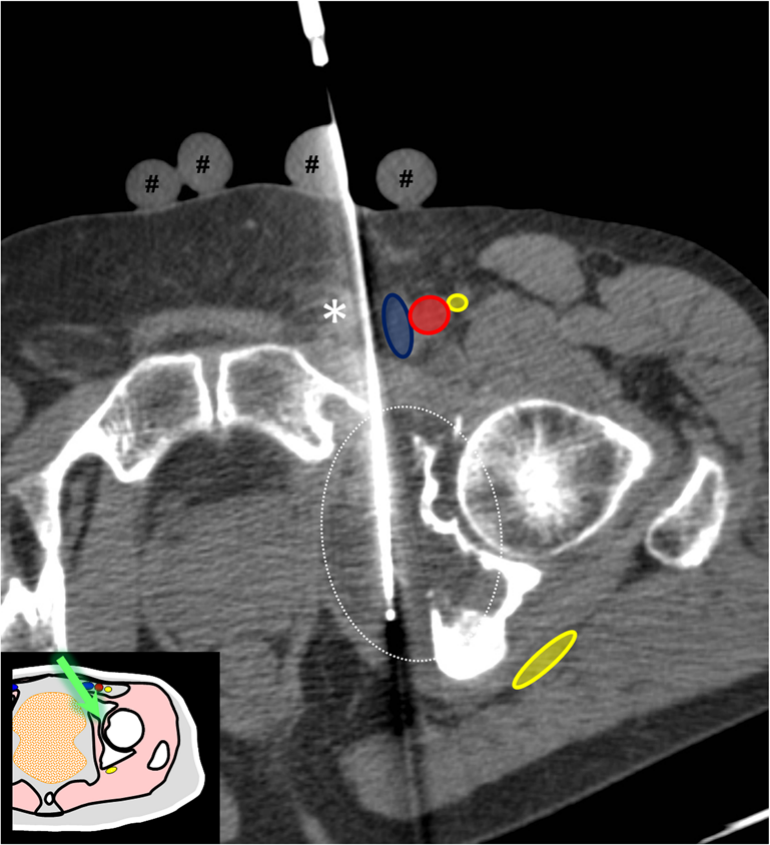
A 68-year-old man with bladder cancer presenting with painful acetabular metastasis. Axial CT shows one of the cryoablation probes inserted in the lesion with an approach between the spermatic cord (*asterisk*) and the femoral vein. The hypodense ice ball (*dotted line*) is seen. Note the glove fingers filled with warm saline over the skin to prevent thermal skin damage (#)
